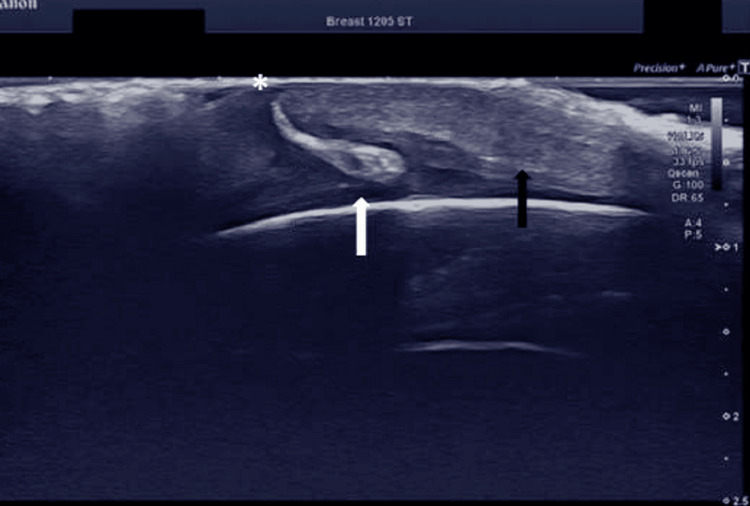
Ultrasound image showing a hyperechoic larval structure (white arrow) embedded in the skin of scalp, surrounded by subcutaneous swelling (black arrow) and a visible entry point (asterisk) at the surface
